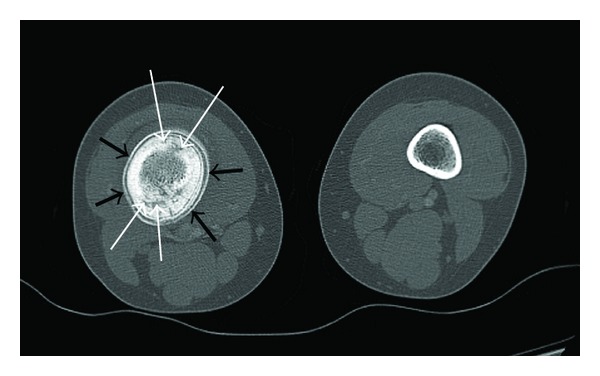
Axial 1.25 mm image enhanced CT through the lesion. The right femoral lesion is well shown on CT, including cortical thickening, cortical lucencies (thin white arrow), and periosteal reaction (black arrows). Note the size discrepancy between the right and left femurs in keeping with the clinically seen asymmetry and fusiform swelling.
